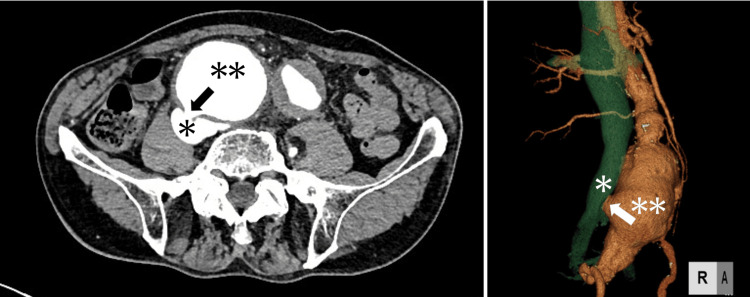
Left: Arterial phase of abdominal contrast-enhanced CT at presentation (horizontal section at the L5 level). Right: 3D-CT reconstruction In the left image, a fistula is visible between the right common iliac artery and the IVC, as the IVC is enhanced during the arterial phase. A single asterisk marks the IVC, while a double asterisk indicates the right common iliac artery (either black or white, depending on the image contrast). The large fistula, caused by rupture of a right common iliac arterial aneurysm (black arrow), led to increased venous perfusion, resulting in high-output heart failure. In the right image, a 3D-CT reconstruction shows the right common iliac artery (red) and IVC (green). The right common iliac artery aneurysm is in contact with the IVC, forming an ACF (white arrow). ACF, arteriovenous fistula; IVC, inferior vena cava
